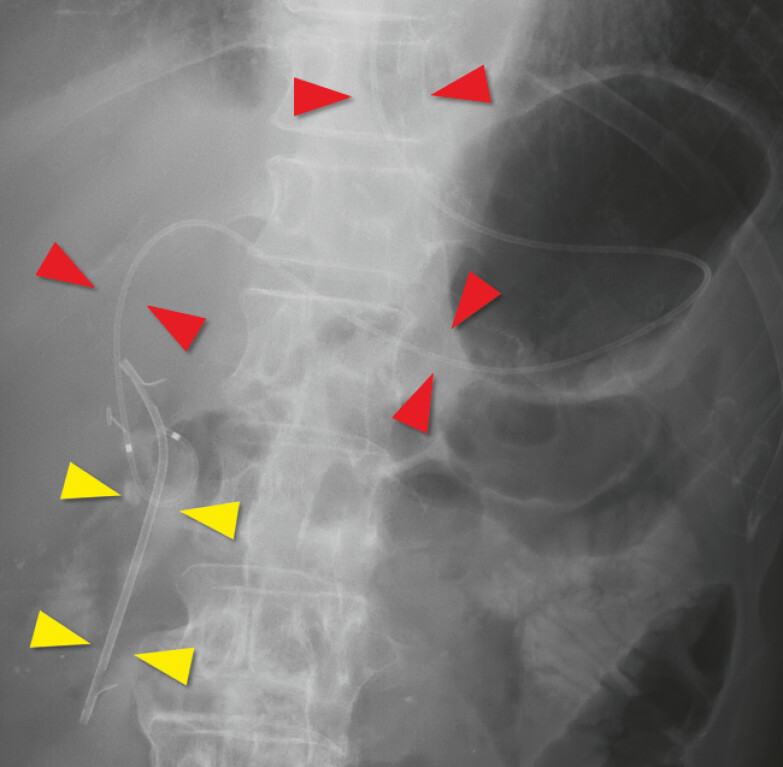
A fluoroscopic image showing an antegrade plastic stent placed across the papilla with its distal end in the duodenum (yellow arrowheads) and an endoscopic nasobiliary drainage tube traversing the endoscopic ultrasound-guided hepaticogastrostomy route (red arrowheads).
